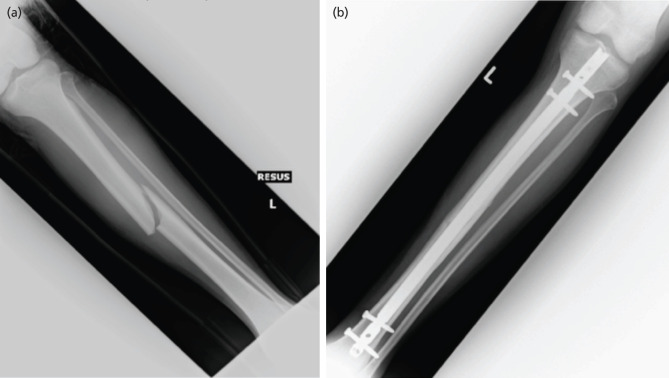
Radiograph images of Case 2. (a) Initial injury on arrival. (b) Post-operative follow-up.
